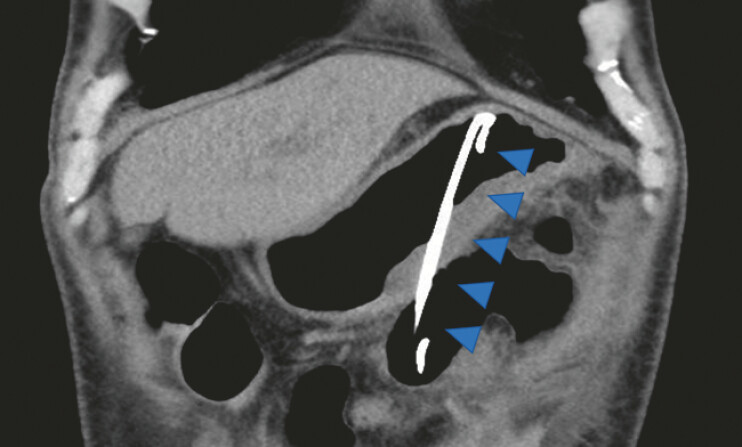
A coronal computed tomography image after the rescue procedure, demonstrating the double-pigtail plastic stent (blue arrowheads) located in the intended trans-gastric position to provide adequate drainage of the pancreatic pseudocyst.
